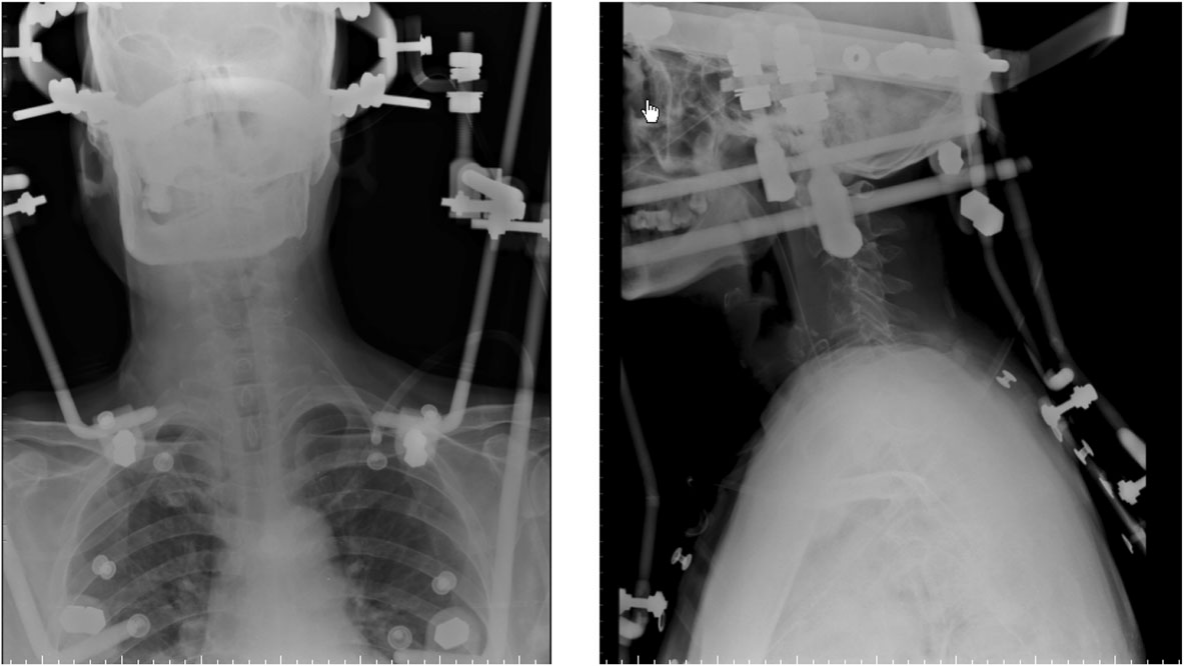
Plain radiograph after application of Hallo frame support
